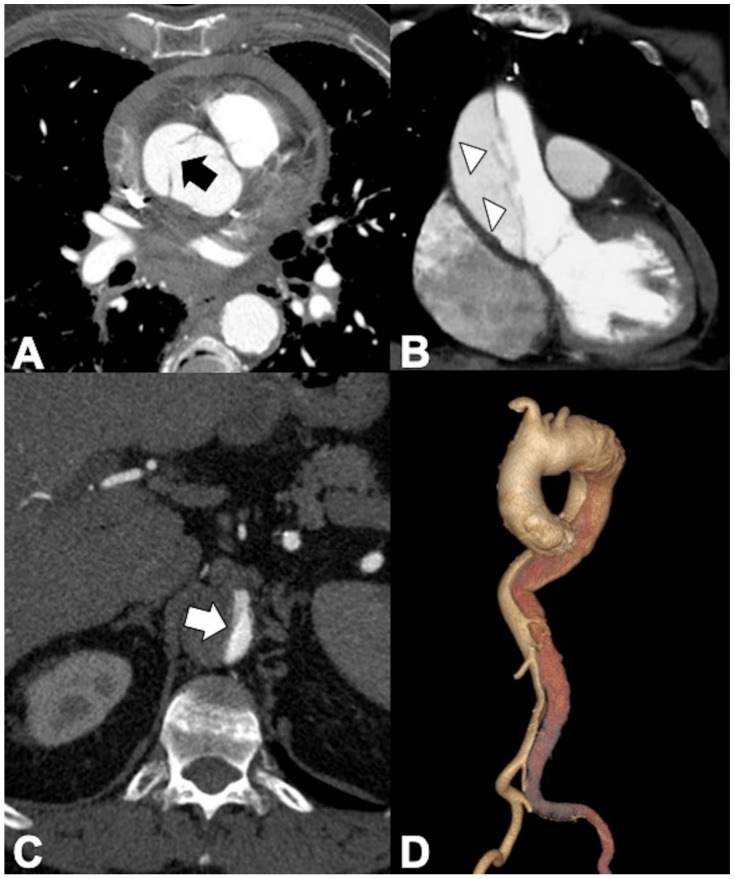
CTA findings in aortic dissection. (A) Axial CTA image showing intimomedial rupture sign in the ascending aorta (arrow) in type A dissection, indicating direction of the intimomedial entrance tear from true to false lumen. (B) Coronal CTA MPR image showing the intimomedial flap travelling parallel to the aorta long axis. Note the cobweb sign by thin, string-like filaments of the media layer in the false lumen (arrowheads). (C) Axial CTA image shows pressure competition between lumina and opacified true lumen collapse (arrow). (D) The different density of the TL (more intensely opacified due to faster flow) and FL in the early angiographic phase allows them to be clearly distinguished in the coronal VR reconstruction.
